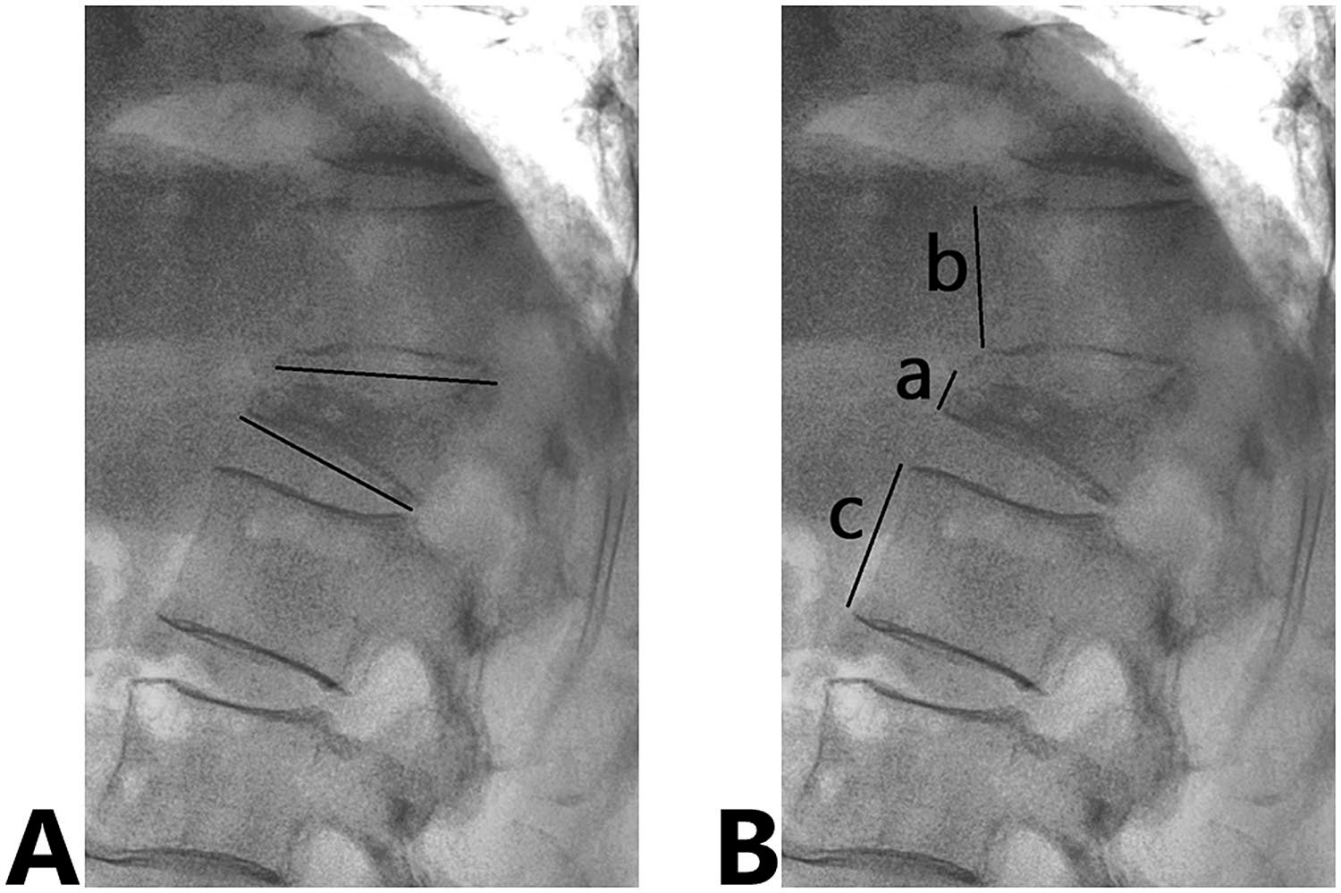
Assessment of the vertebral deformity. (A) The vertebral wedge angle was measured from the lateral view of radiograph in weight-bearing position. (B) The percentage vertebral body height was calculated by the following formula: (2 a/[b + c] × 100.
